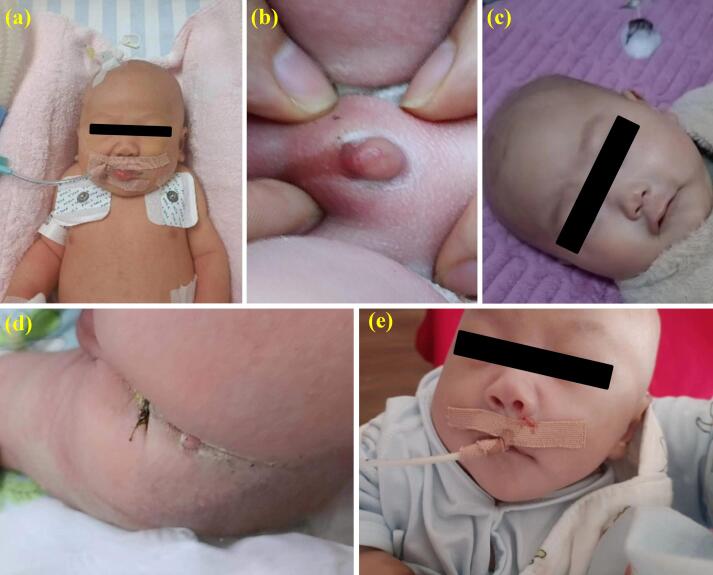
Laboratory investigations, including complete blood count, biochemical parameters, blood amino acids, urine organic acids, and cultures of blood and sputum, revealed no significant abnormalities. Ultrasound imaging indicated the presence of an atrial septal defect measuring approximately 2.4 mm, potential hypoplastic testicles, and laxity in the left hip joint. CT scans revealed nasal malformations, specifically left choanal atresia and right posterior nasal stenosis, which were further confirmed by fiberoptic bronchoscopy and cranial MRI. Additionally, distal enlargement of the right 6th and 7th ribs was noted on CT imaging (Figure 2).
